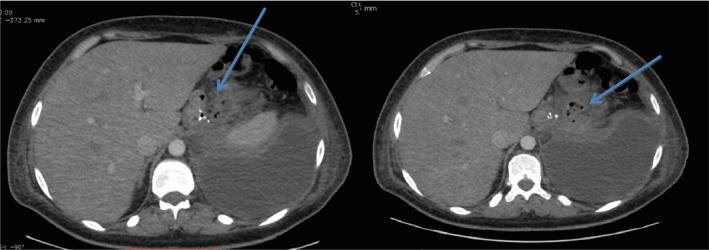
CT scan abdomen showing left upper quadrant staple line leak corresponding to the gastro‐colic fistula (arrows point to fistulous tract), note the left pleural effusion.
